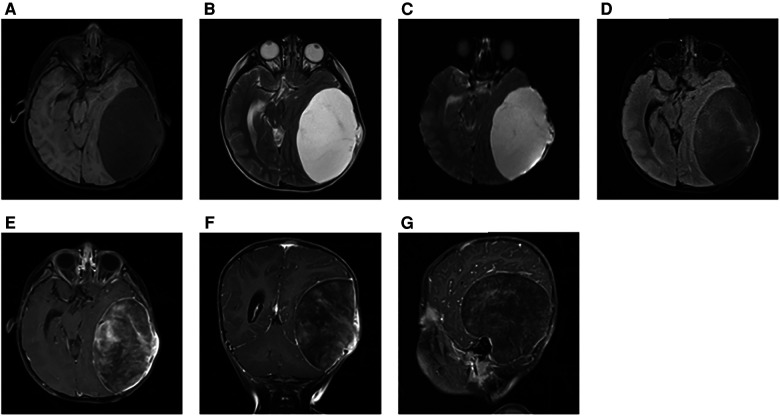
At the first illness, on T1-weighted and T2-weighted images, the tumor revealed long signal abnormal signals (A,B). In FLAIR, it was an iso-low signal (C). DWI showed a low-signal tumor (D). Contrast-enhanced MRI images showed a markedly enhanced mass (E–G).
